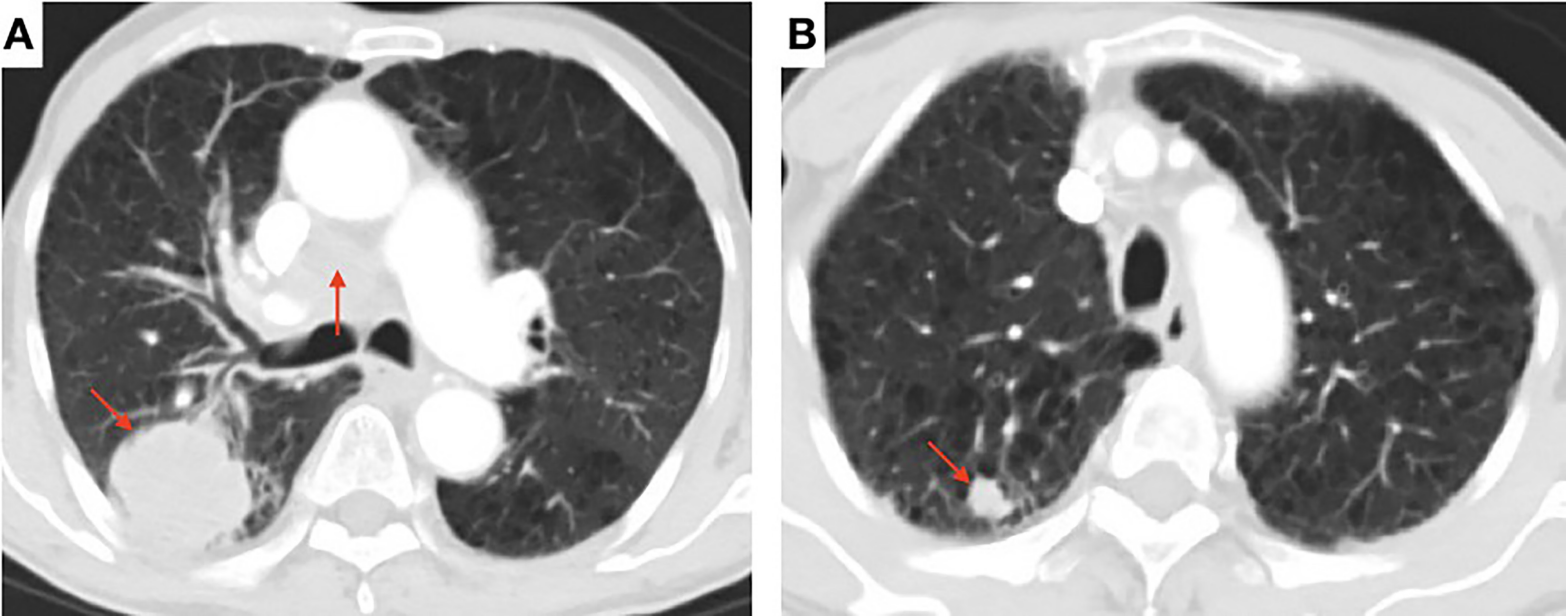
Contrast-enhanced chest computed tomography (CT) scan showing (A) a soft mass (45 × 43 mm) in the posterior segment of the right upper lobe and the enlarged right hilar and mediastinal lymph nodes (arrow) and (B) multiple pulmonary nodules with a maximum diameter of 20 × 14 mm (arrow).
